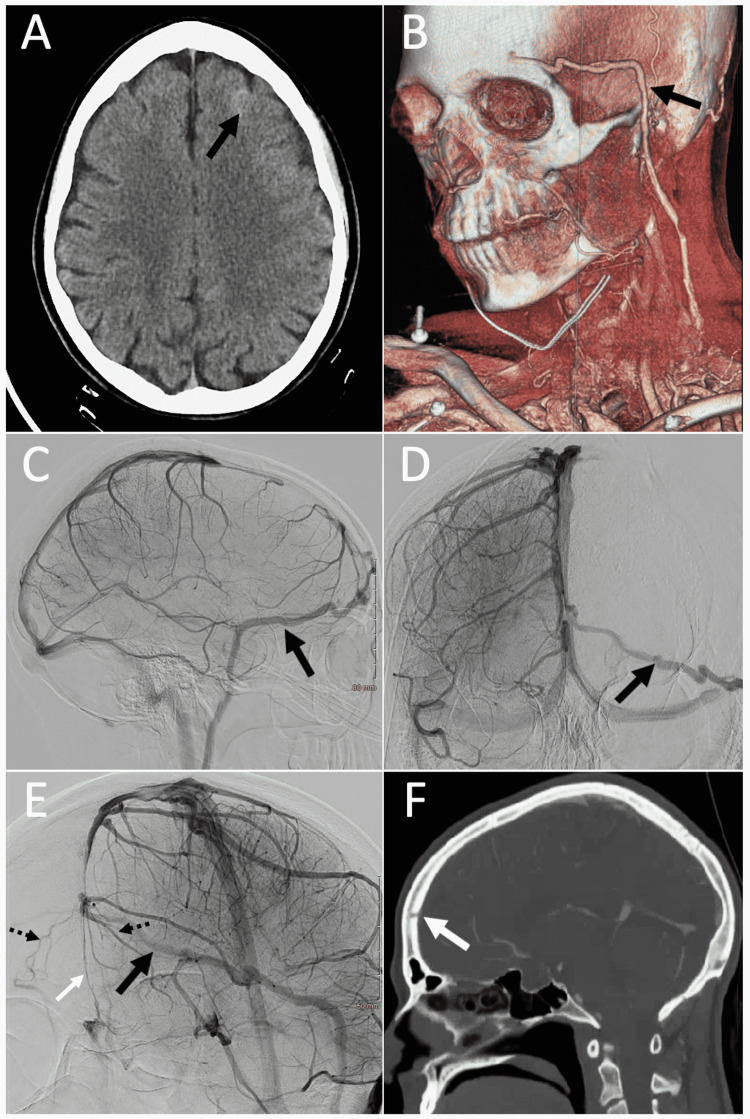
Imaging findings of the case presentation (A) Computed tomography (CT) scan showing left frontal subarachnoid hemorrhage (SAH) (black arrow). (B) 3-D imaging showing the exit of the anomalous vein through a foramen in the left frontal bone and then traveling superficially toward the neck. (C) Lateral projection of the right internal carotid artery angiogram showing the anomalous draining vein (black arrow). Note the hypoplastic anterior portion of the superior sagittal sinus. (D) Anterior-posterior projection of the right internal carotid artery angiogram showing the anomalous vein traversing from the right to the left side (black arrow). (E) Oblique projection of left internal carotid artery angiogram showing the anomalous vein (large black arrow) and its independence from the superior sagittal sinus notable more posteriorly. Additionally, the patient was noted to have a patent vein of foramen caecum (white arrow) and two smaller veins draining the bilateral orbital area into the venous confluence, then drained by the large anomalous frontal vein (dotted black arrows). (F) CT angiogram bone window demonstrating the abnormal osseous channel in the left frontal bone (white arrow).
